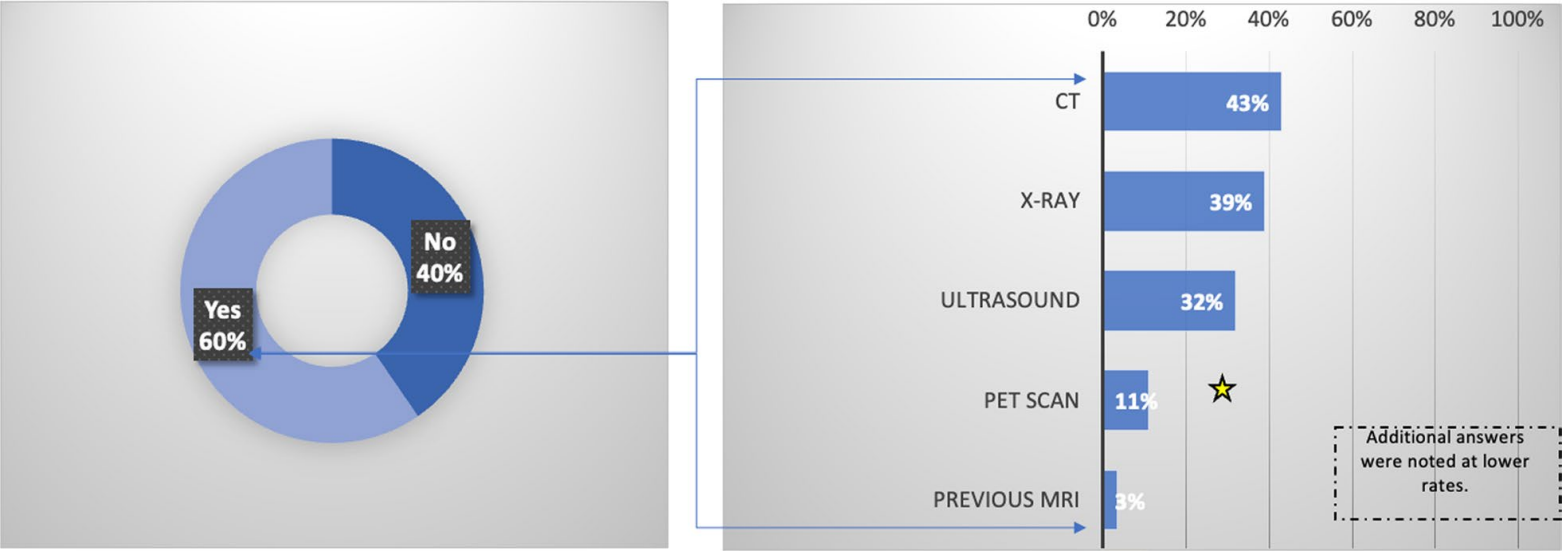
Percentage (%) of n = 557 that underwent a preliminary test prior to an MRI examination for the same problem, and the respective breakdown of imaging modality employed
